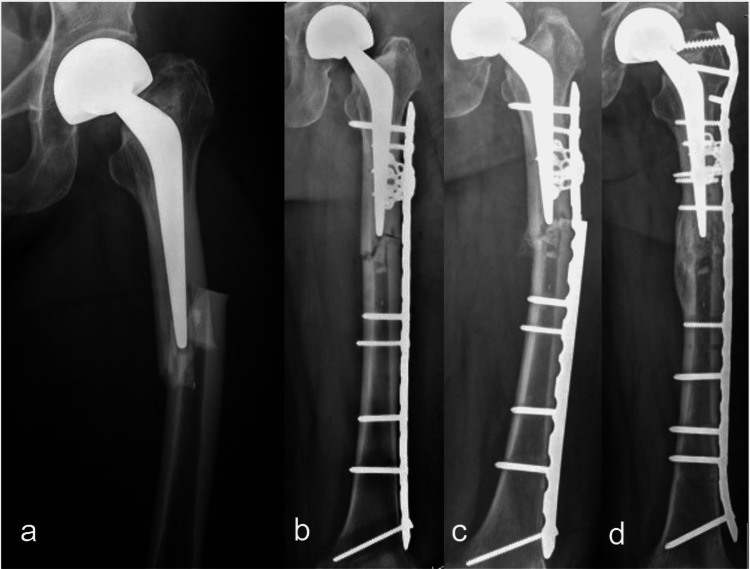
The radiographs from Case 4 a) complete transverse fracture with medial spike with clinical thigh pain at presentation, b) immediate post-operative ORIF with plate and screws with locking attachment plate, c) plate breakage with varus malalignment of the fracture four months after the fixation (clinical pain after re-falling) d) fracture healing consolidation at seven months after the first operation (union at three months after the second operation).
